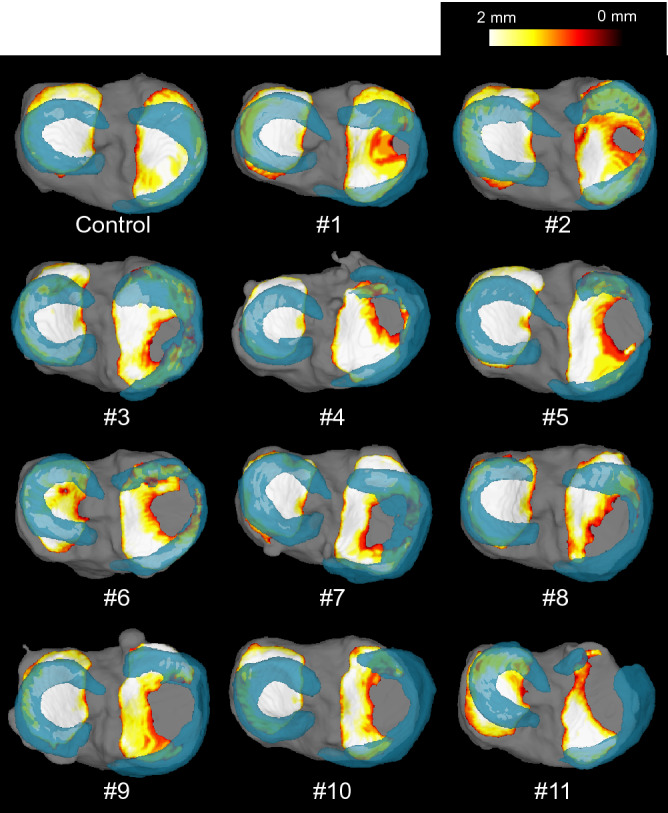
Superimposition of the meniscus on the cartilage thickness mapping of the tibia by 3D MRI. The thickness of the cartilage is indicated by color mapping, where white indicates a thickness ≥ 2 mm and the progression from yellow to red indicates thinning of the cartilage. The meniscus is shown in translucent blue, and the tibia bone is shown in gray.
